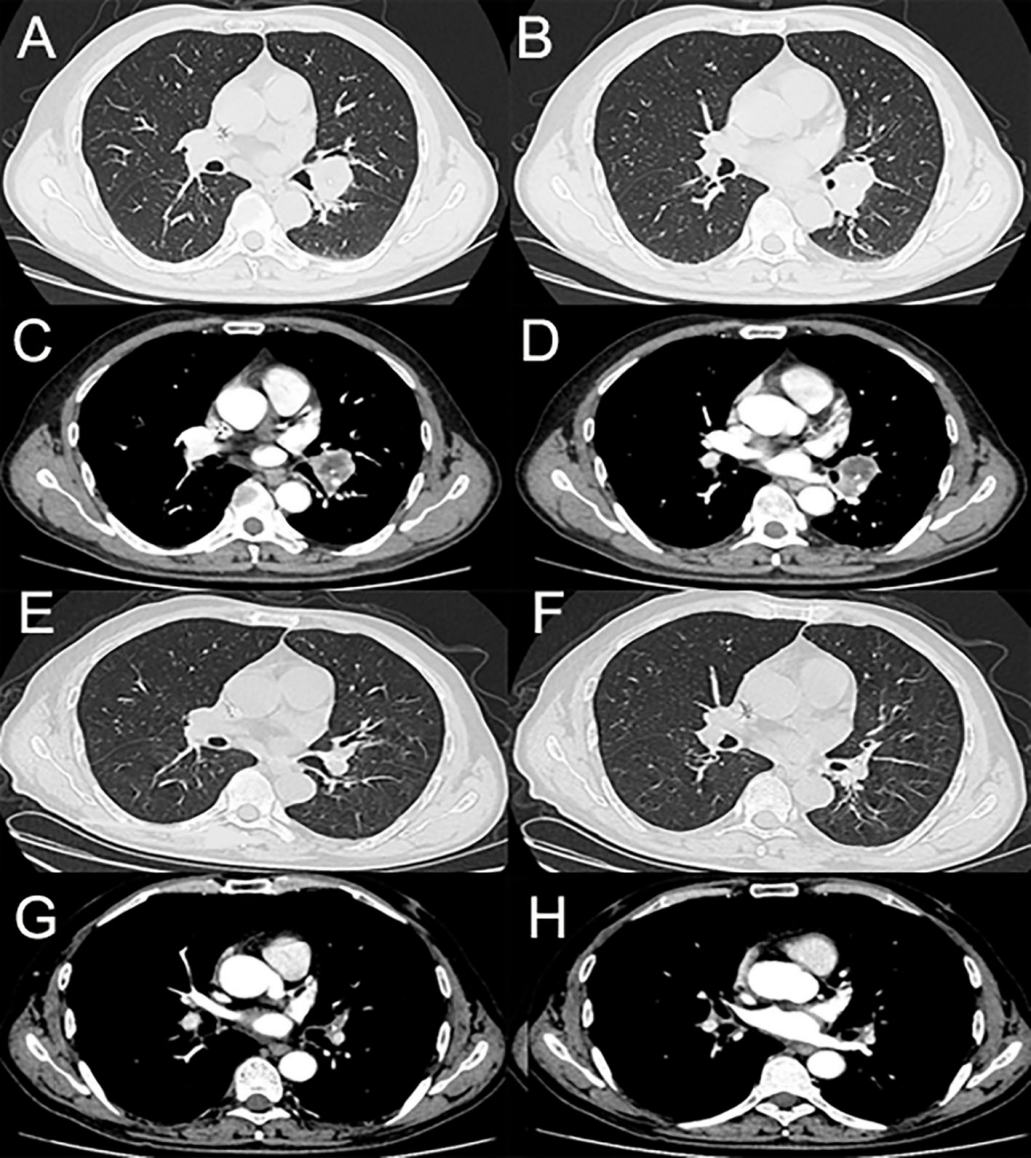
(A–D) The enhanced computed tomography (enhanced-CT) scans of the tumor before neoadjuvant therapy. (E–H) The enhanced-CT scans of the tumor after three cycles of neoadjuvant therapy.
